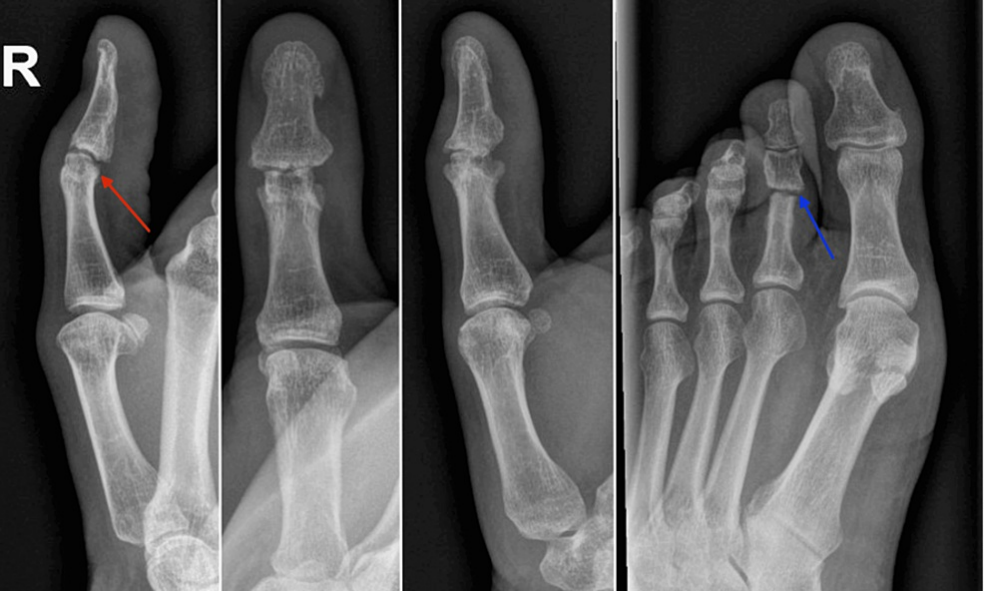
Three-month post-operative radiographs showing a well-healed osteochondral bone graft without resorption. On the lateral view, a small volar bony lip can be seen, which limits complete flexion (red arrow). The second toe donor site developed a pain-free fibrous union (blue arrow).
